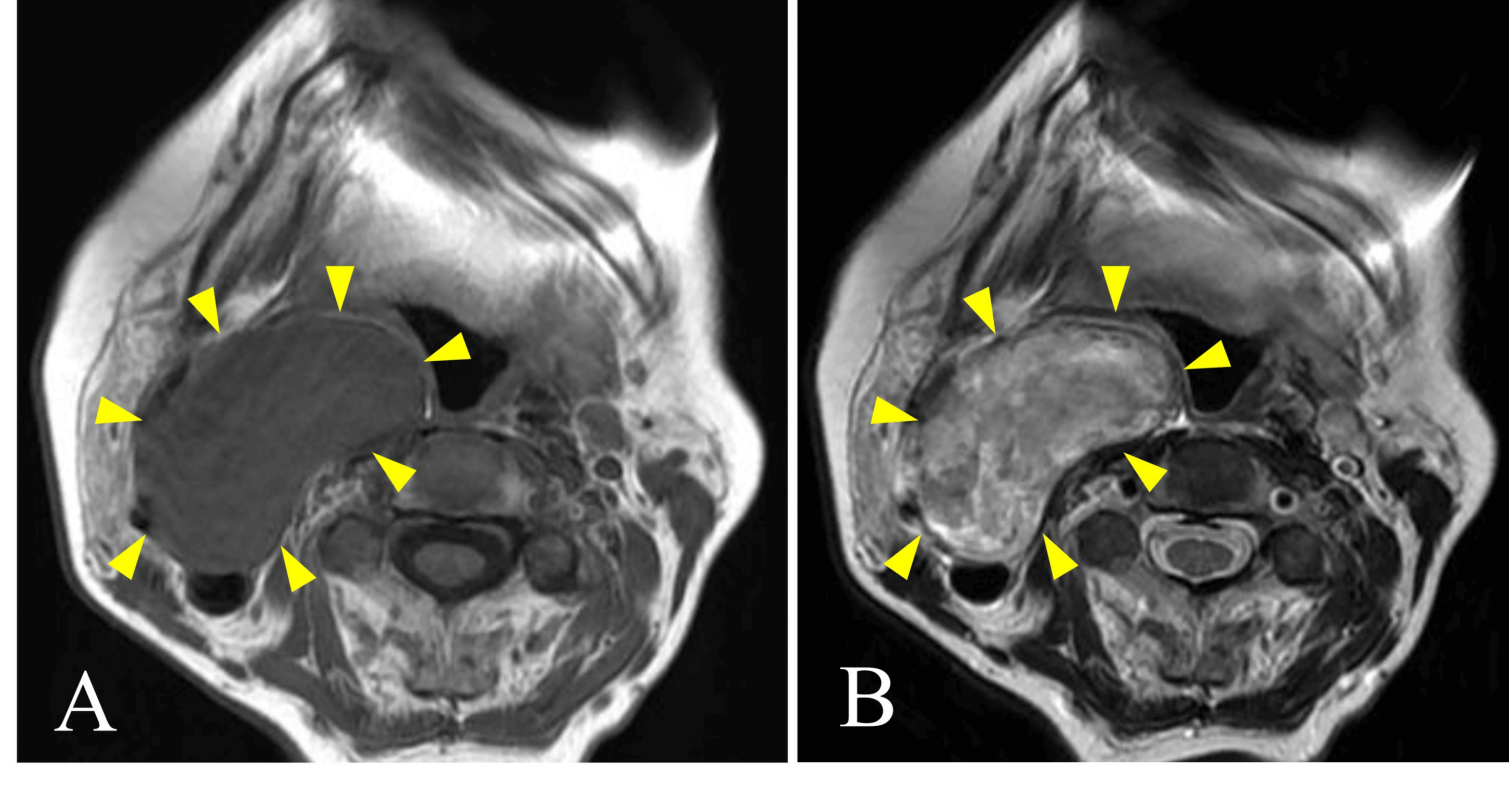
MRI images: (A) T1-weighted image; (B) T2-weighted image. A mass with isointensity on the T1-weighted image and heterogeneous high intensity on the T2-weighted image was seen in the right parapharyngeal space. The border was clear.
